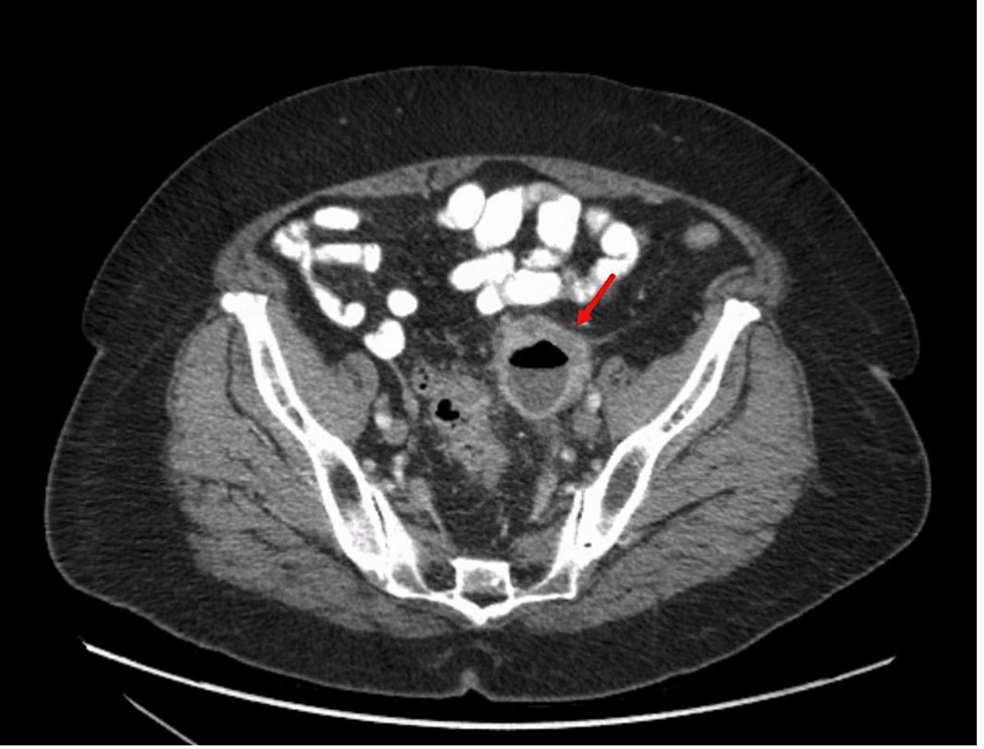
Follow-up CT abdomen pelvis with contrast image after three months showing persistent but slightly decreased abdominal abscess. CT, computed tomography
